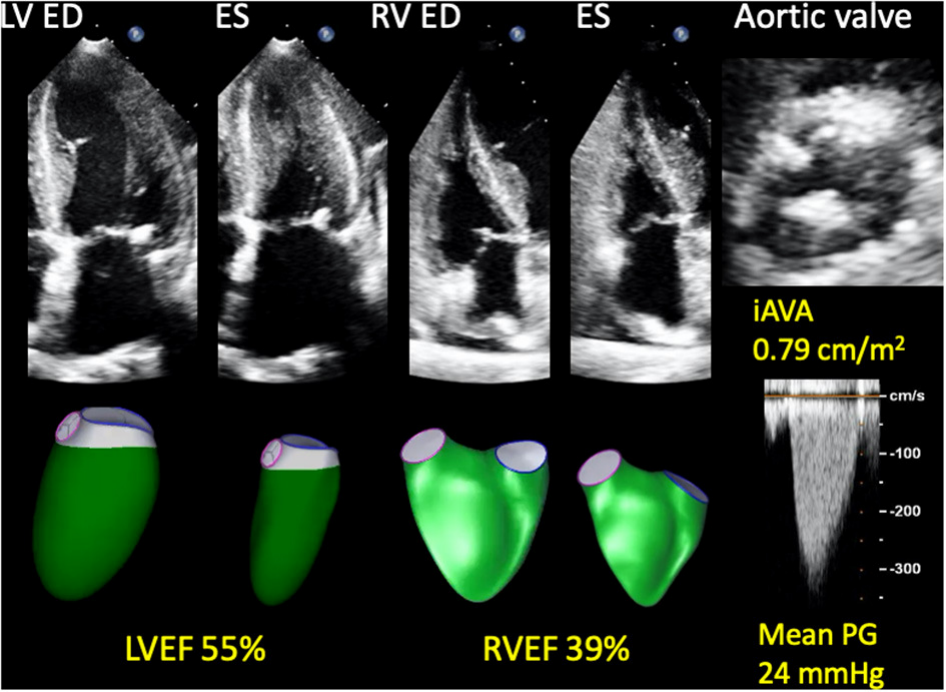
Representative 3D RV and LV analyses in a patient with asymptomatic AS who developed a cardiac event. A 74-year-old woman who developed heart failure three months after baseline echocardiography. AS was moderate and LVEF was preserved; however, RVEF was reduced. She also had hypertension, chronic kidney disease, and chronic obstructive pulmonary disease. 3D, three-dimensional; AS, aortic stenosis; ED, end-diastole; ES, end-systole; iAVA, indexed aortic valve area; LV, left ventricular; LVEF, left ventricular ejection fraction; PG, pressure gradient; RV, right ventricular; RVEF, right ventricular ejection fraction.
